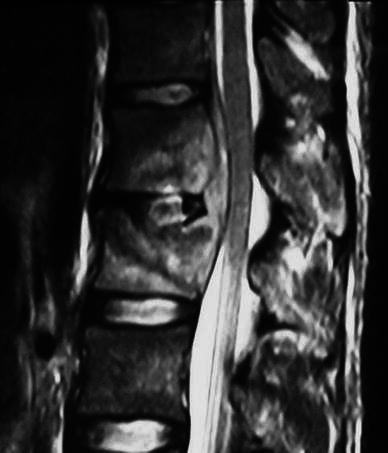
T2-weight magnetic resonance image, sagittal view. Involvement of T12 and L1 with progressive narrowing of the spinal canal
